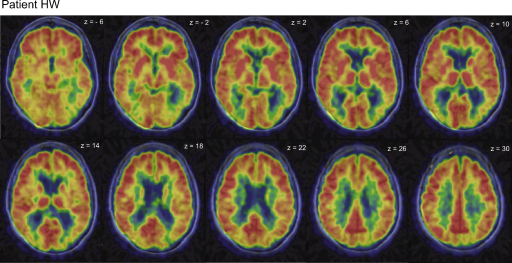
18-Fluorodeoxyglucose positron emission tomography (FDG-PET) scans overlayed with the anatomical magnetic resonance imaging (MRI) scans for patient HW reveal reduced metabolism in the parieto-temporo-occipital cortex bilaterally; see main text.
